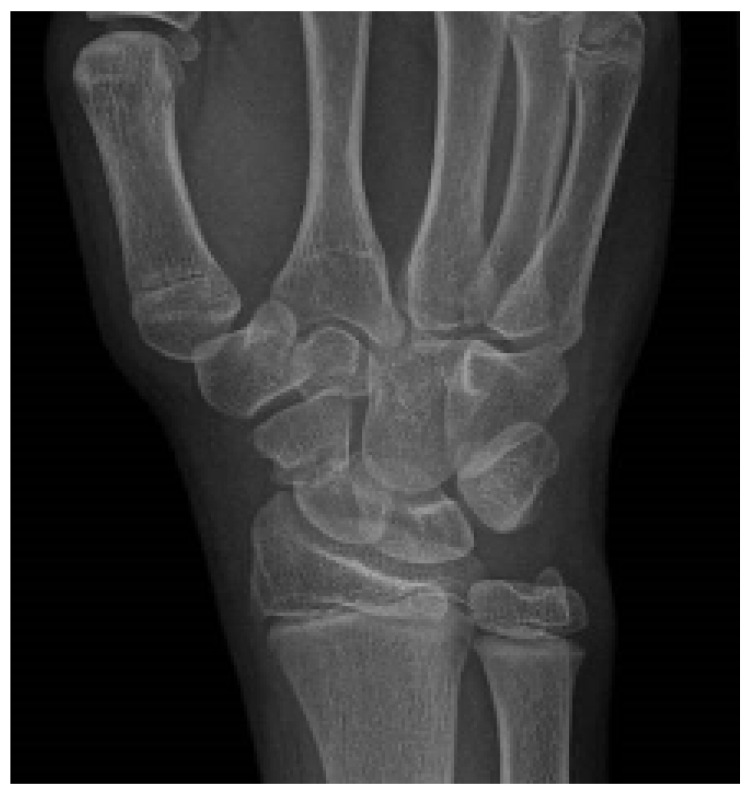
Radiographic evidence of scaphoid waist non-union in Patient 2.
